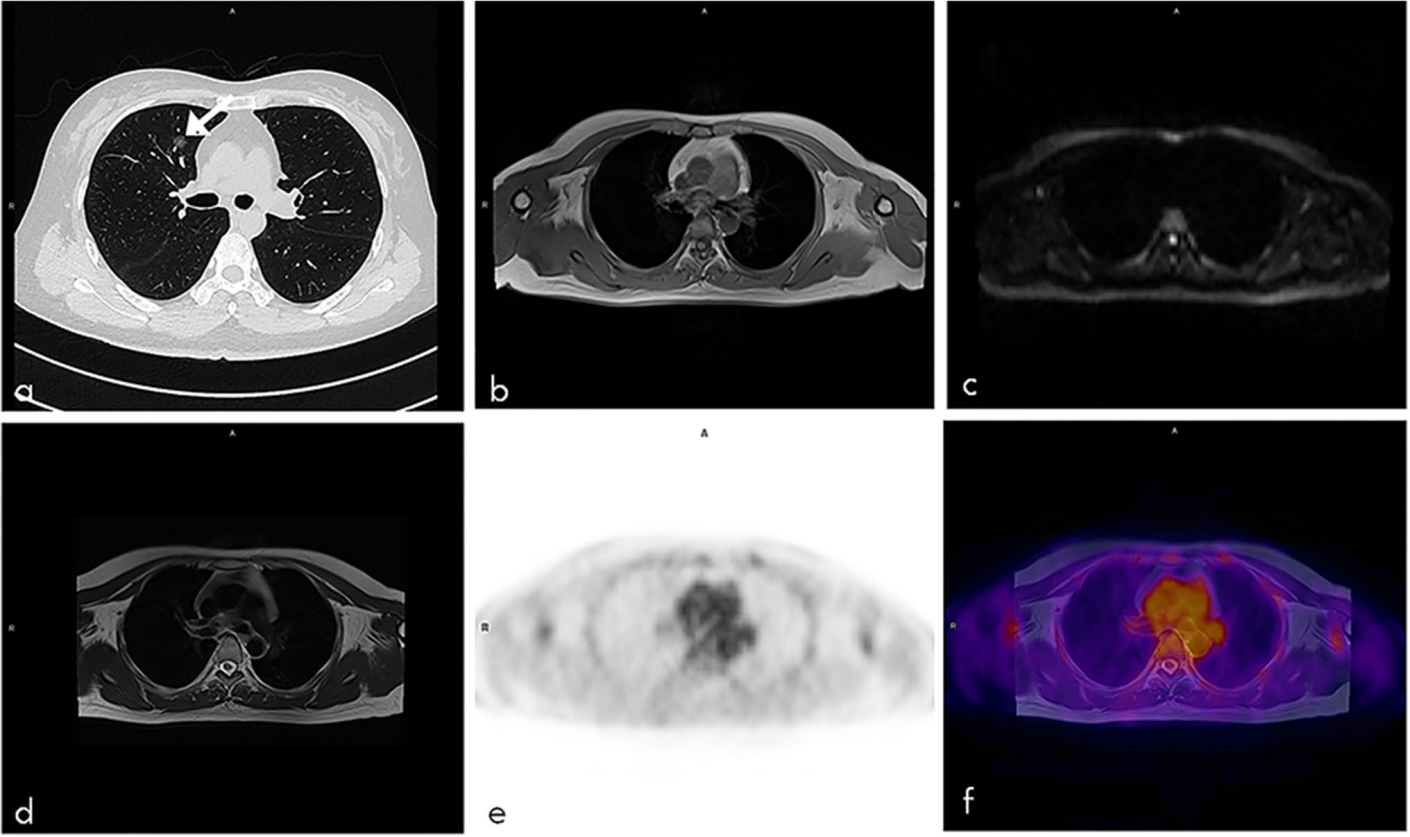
Axial CT and PET/MRI images of a 48-year-old woman with lung cancer. A ground-glass density nodule (arrow) is seen in the anterior upper lobe of the right lung with a size of 1.0 × 0.8 cm (a), which is negative on the T1-weighted image (b), diffusion-weighted image (c), T2-weighted image (d), PET (e), and fused T2-weighted and PET image (f). Pathology confirmed to be adenocarcinoma
